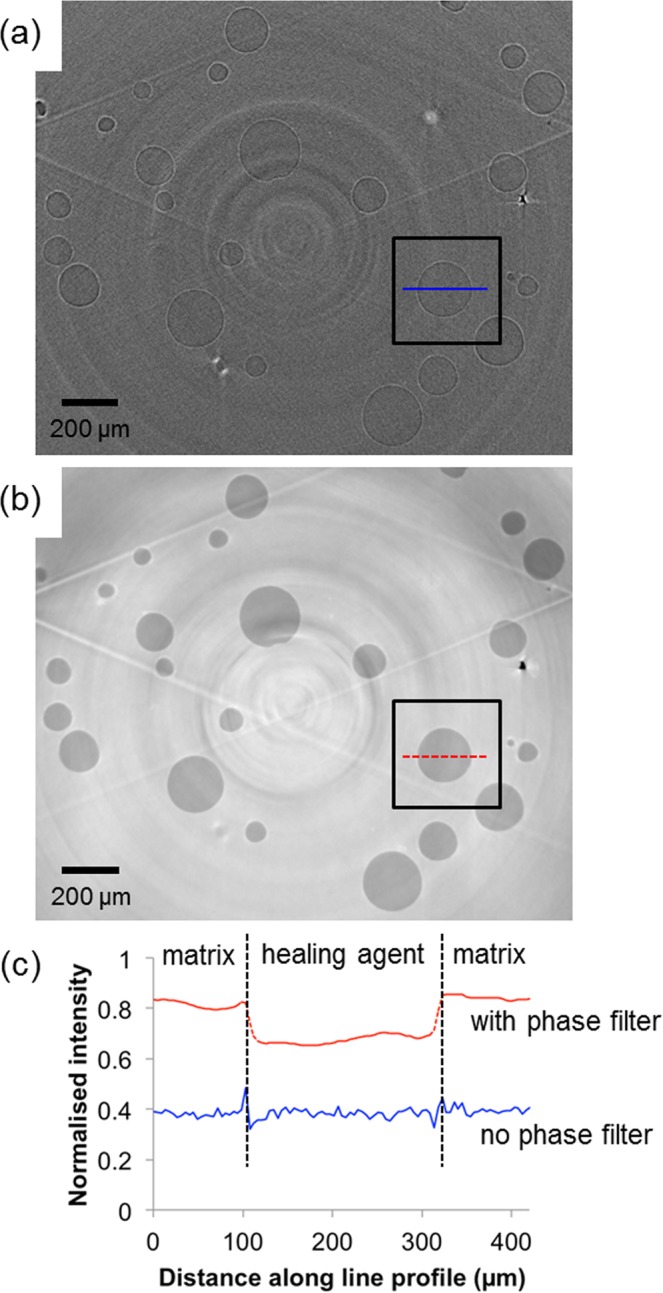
Demonstrating the application of phase contrast X-ray imaging. A virtual horizontal (*x-y*) slice through the reconstructed 3D volume of the microcapsule-based self-healing epoxy sample, shown (a) without phase backpropagation filtering and (b) with phase backpropagation filtering. (c) Line intensity profiles taken across the capsule indicated in (a,b), demonstrating the effect of the filter.
